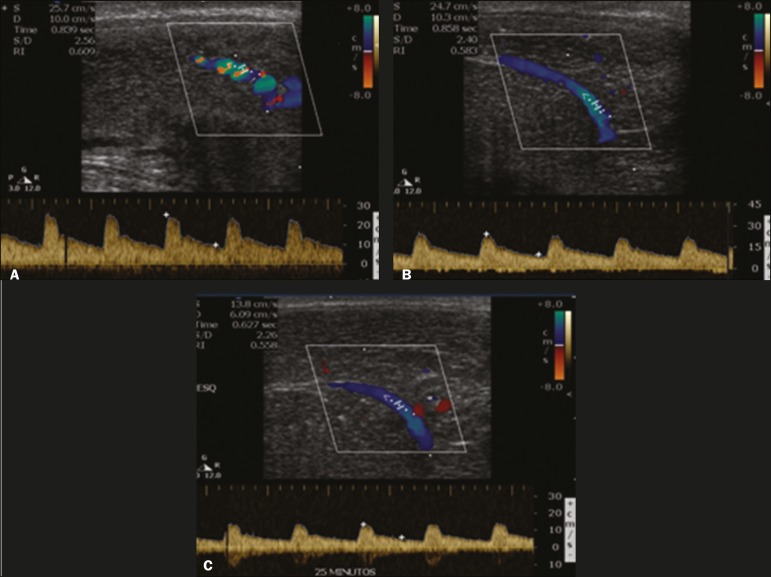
Longitudinal, ventral ultrasound of the penis, with pulsed mode and color
